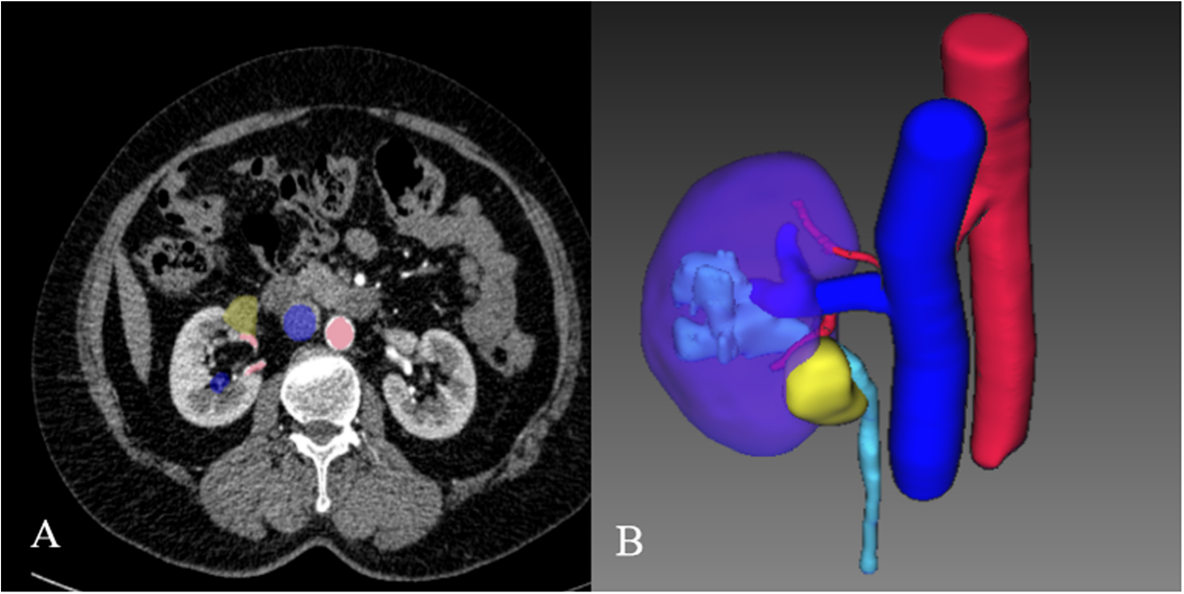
Renal hilar tumor’s 3D reconstruction and traditional CT scans. a General CT scans; red reflects artery, blue reflects vein, and yellow reflects tumor. b The reconstructive urinary system which include lesion, kidney, collective system, and blood vessels
